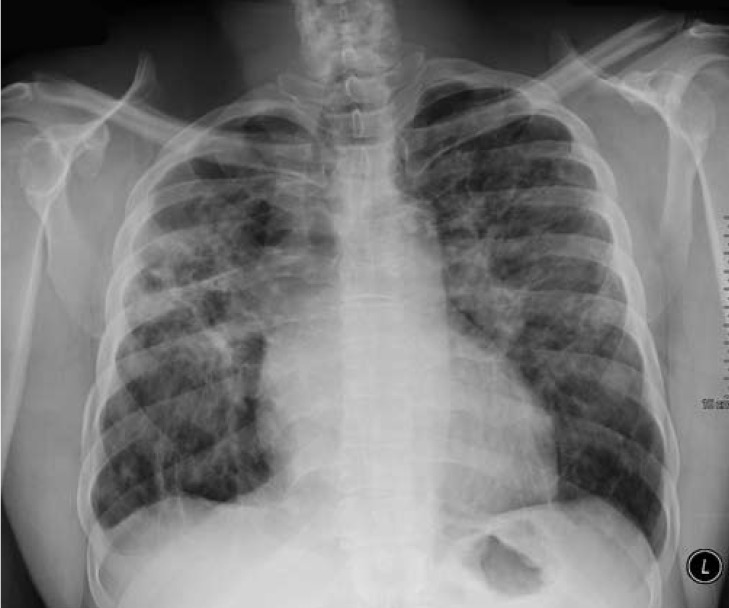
Plain film of the chest demonstrating extensive interstitial lung disease and peri-hilar consolidations versus increased pulmonary vasculature.
